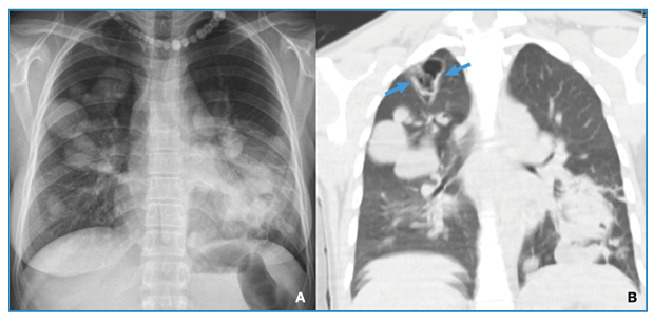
(A) Emergency posteroanterior chest radiograph showing diffuse cystic lesions in both lungs. (B) Thoracic CT scan depicting a ruptured cavitary lesion in the right apical upper lobe with evacuated intracystic fluid and visible fragments of the germinal membrane, which are most likely the source of hydatoptysis (blue arrows indicate the fragments).
